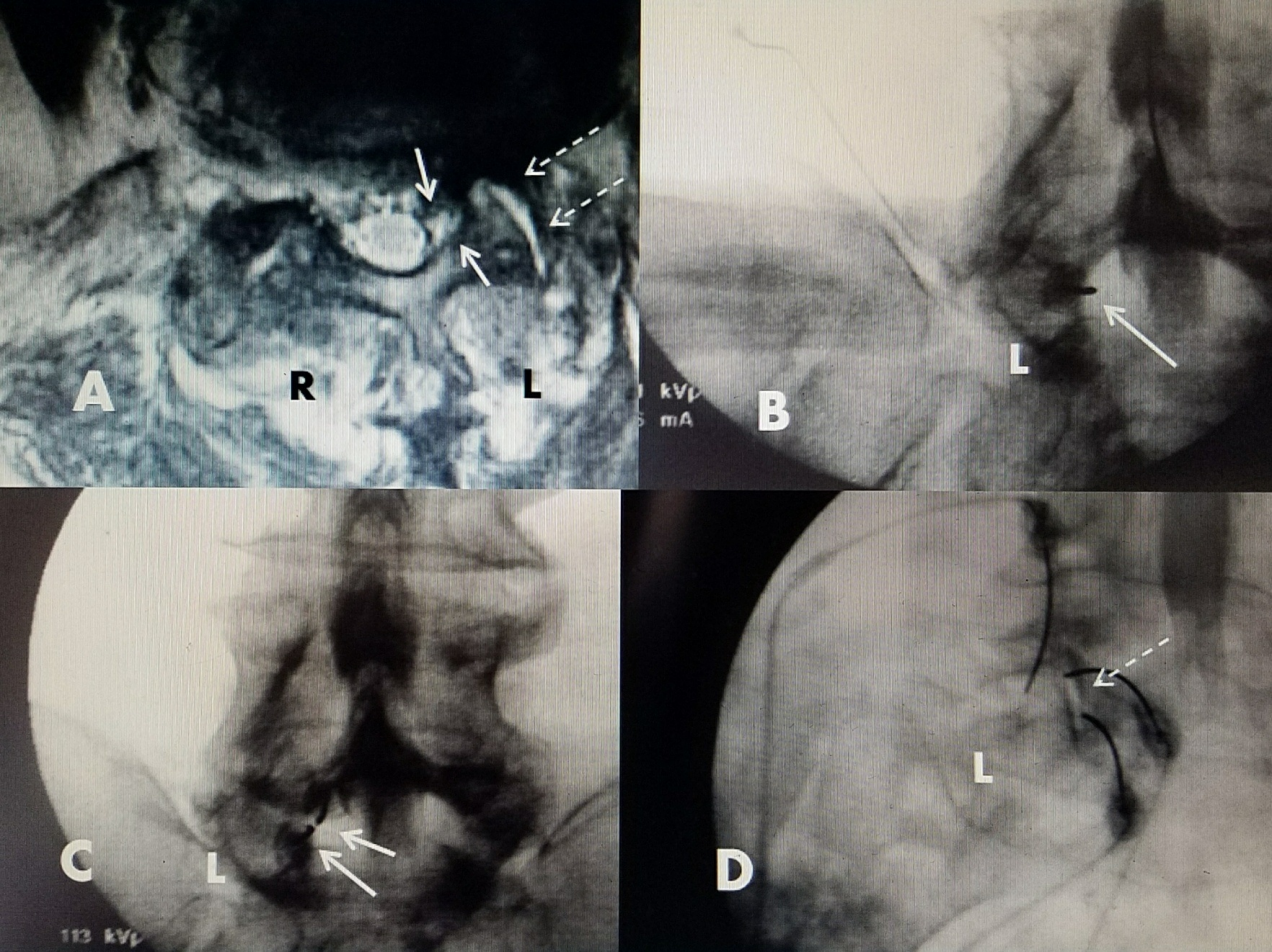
Electrode placement for L5-S1 left facet cyst Left side (L) on intraoperative fluoroscopy. Left side (L) and right side (R) on axial MRI scan. A: Axial T2 MRI showing cyst medial to facet on left side (L) in lateral recess (solid white arrows). High intensity fluid in adjacent facet joint (dashed white arrows). B: Intraoperative film with myelogram contrast showing defect to left (L) corresponding to location of cyst seen on MRI in (A). First RF electrode directed into facet cyst (solid white arrow). C: Second RF electrode placed in cyst below the initial RF electrode (solid white arrows). D: Oblique film showing RF electrodes in L5-S1 facet joint and capsule (dashed white arrows).
